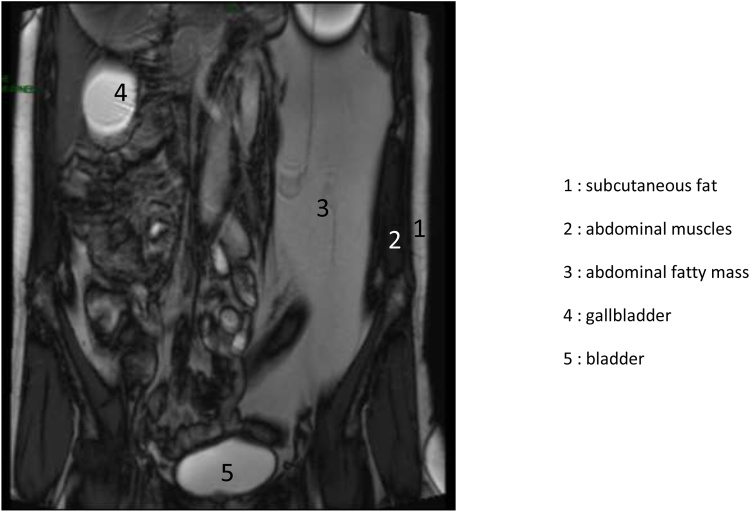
Coronal cut showing the lipomatous lass of the left mesocolon (MRI) in T2 hypersignal.
